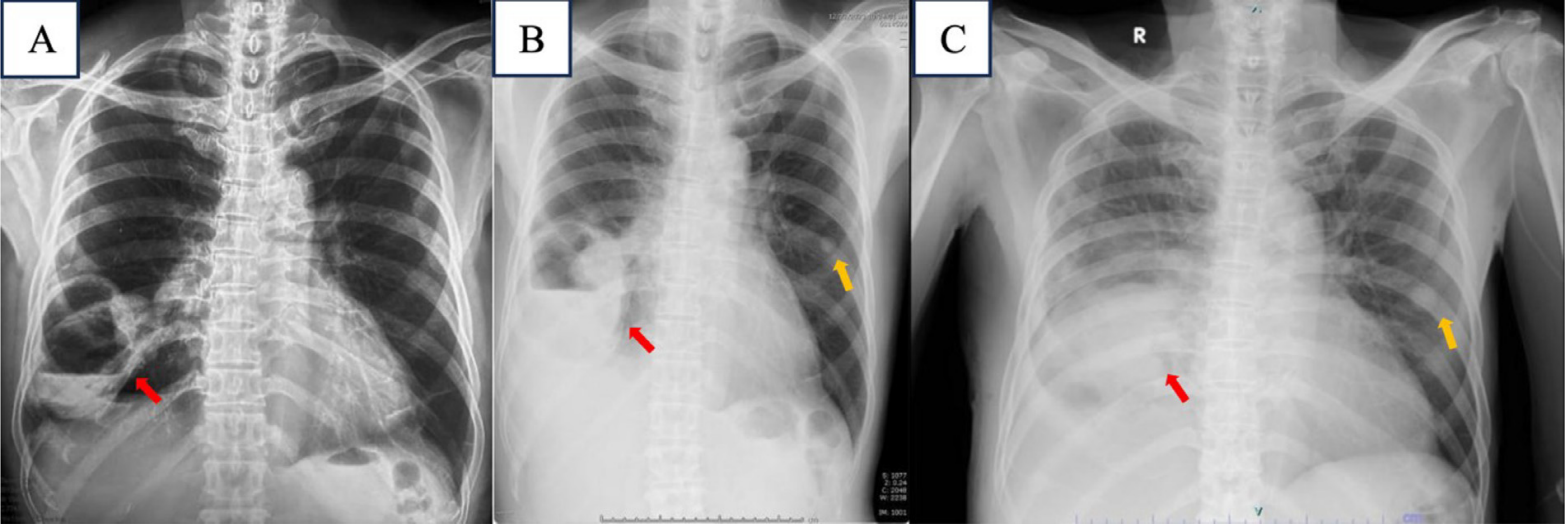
(A) Initial chest X-ray shows there is cavitating lesion with air-fluid level inside it (red arrow) (B) Second chest X-ray. The size of the lesion remains constant, but there is also right pleural effusion and nodule in left parahilar (yellow arrow) (C) and third chest X-ray before open biopsy shows, there is increase fluid level in the cavity and nodule in left parahilar (yellow arrow).
